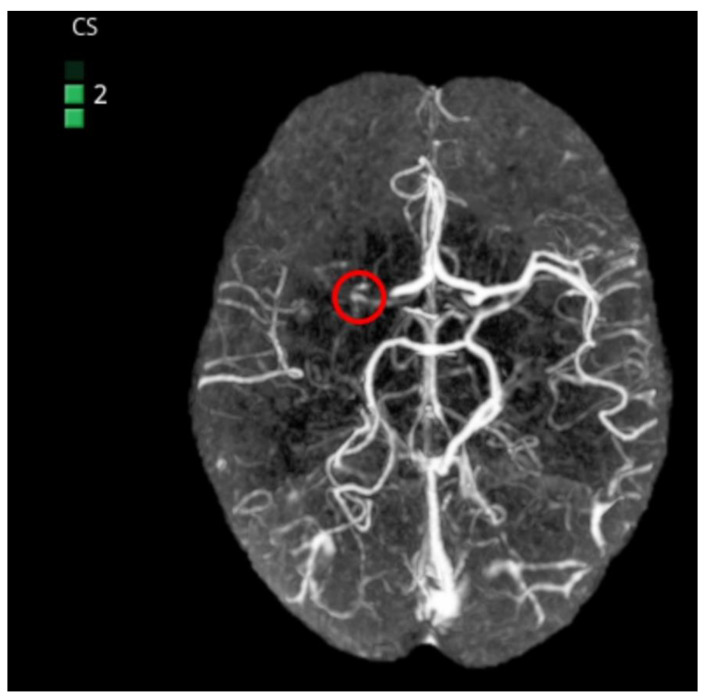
Detection of right middle cerebral artery occlusion by artificial intelligence from CTA. Collateral score = 2.
